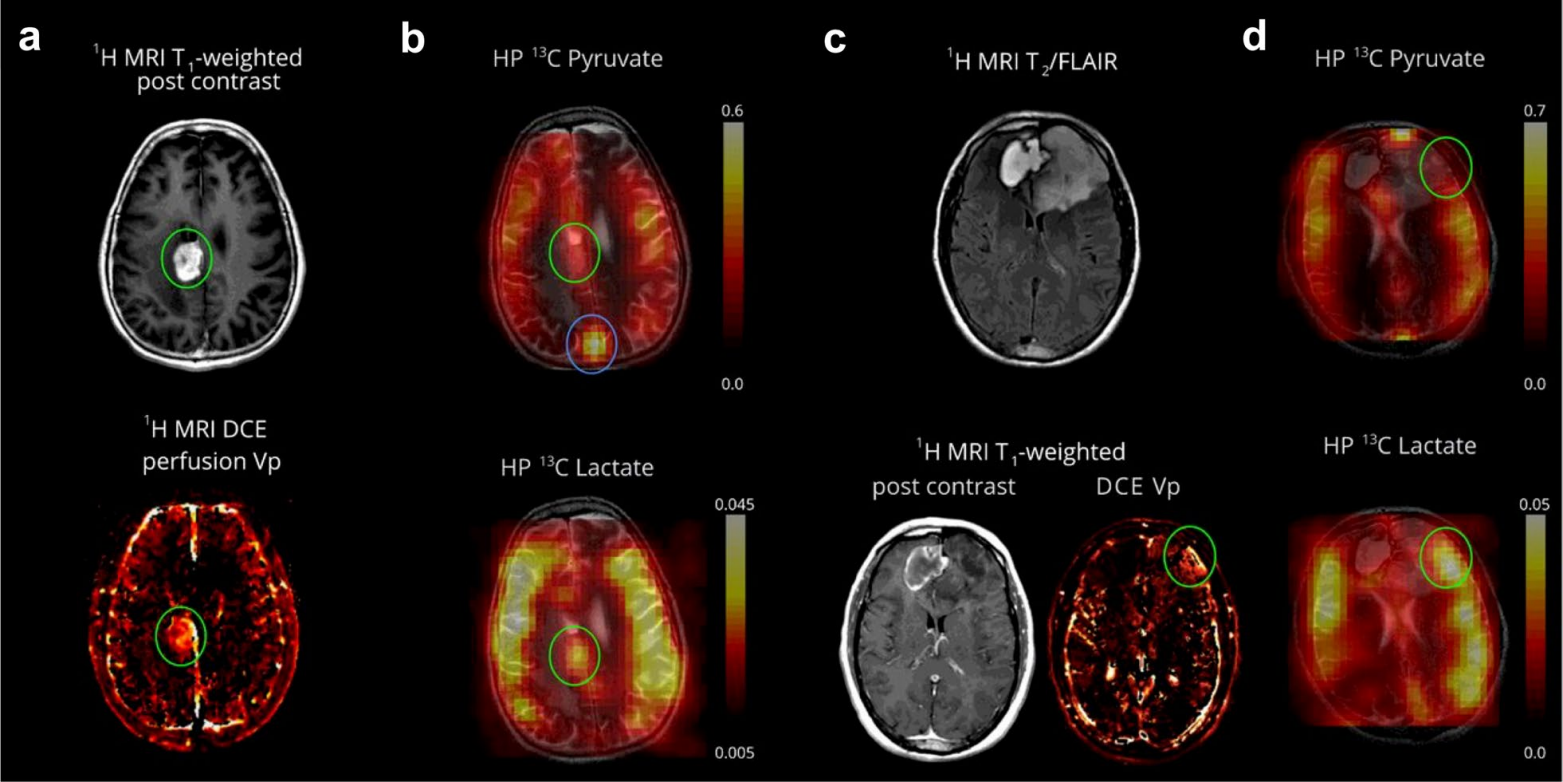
Pyruvate metabolism in a recurrent glioblastoma and treatment-related changes (Patient 2, a and b) and anaplastic oligodendroglioma (Patient 1, c and d). An enhancing mass is located in the right cingulate gyrus (green oval) (a). The hyperpolarized pyruvate map shows high signal corresponding to the cortex/juxtacortical regions and superior sagittal sinus. The volume normalized pyruvate signal in the lesion is 1.3-fold higher than the entire brain. The HP lactate map shows mildly elevated signal corresponding to the lesion, similar to background lactate production by the brain. The volume normalized lactate signal in the lesion is 1.0-fold of the lactate over the entire brain (cortex and white matter) (b). The T2-FLAIR image shows an expansile left frontal mass and contracting right frontal hematoma. The left frontal mass has minimal, patchy enhancement and a small component of elevated plasma volume (green oval) visualized by DCE MRI (c). The HP pyruvate map shows high signal corresponding to the cortex/juxtacortical regions and superior sagittal sinus, but relatively lower signal corresponding to the small hyperperfused component. The HP lactate map shows mildly elevated signal corresponding to the hyperperfused component, similar to background lactate production by the brain (d). Cancer Res. 2018;78(14):3755–3760
